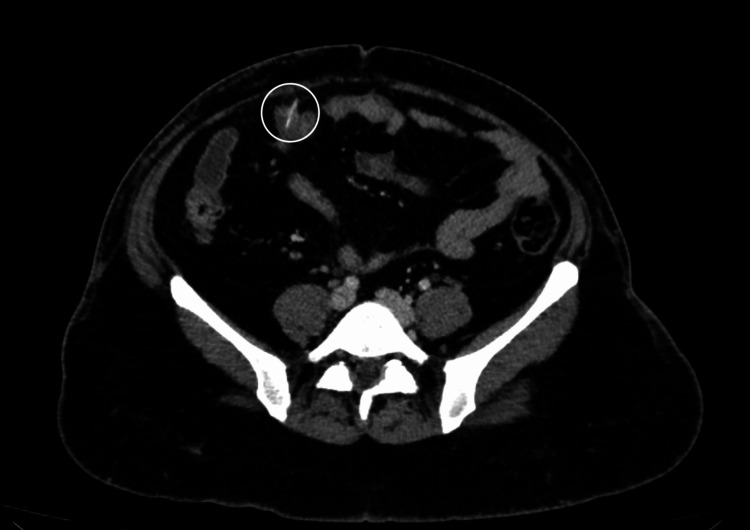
Foreign body identified on CT scan, in the ileal loops, with surrounding area of inflammation (white circle)
